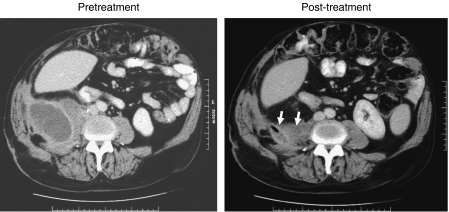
Computed tomographic scan of the abdomen at baseline and after the full treatment in a patient with initially progressive disease that shows a reduction in size of the retroperitoneal collection (arrows).
